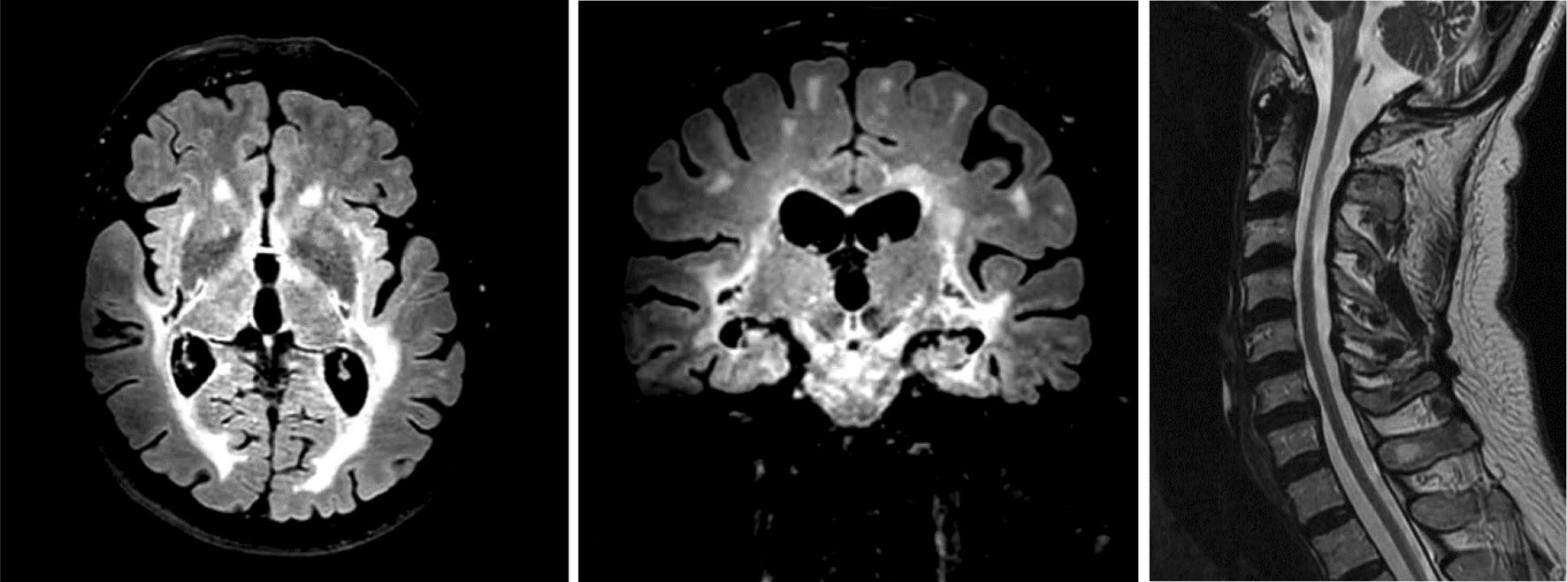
Cranial and spinal magnetic resonance imaging sections and MRI sequence from left to right—axial FLAIR, coronal FLAIR, and sagittal T2
